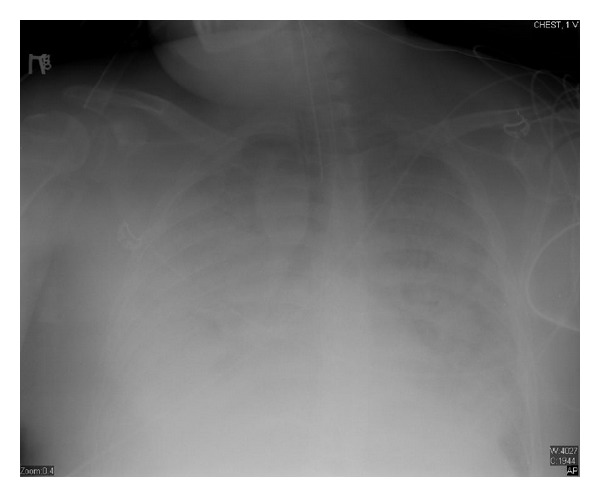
Chest X-ray day 22 after chemotherapy (ANC was 11,500 cell/*μ*L).
